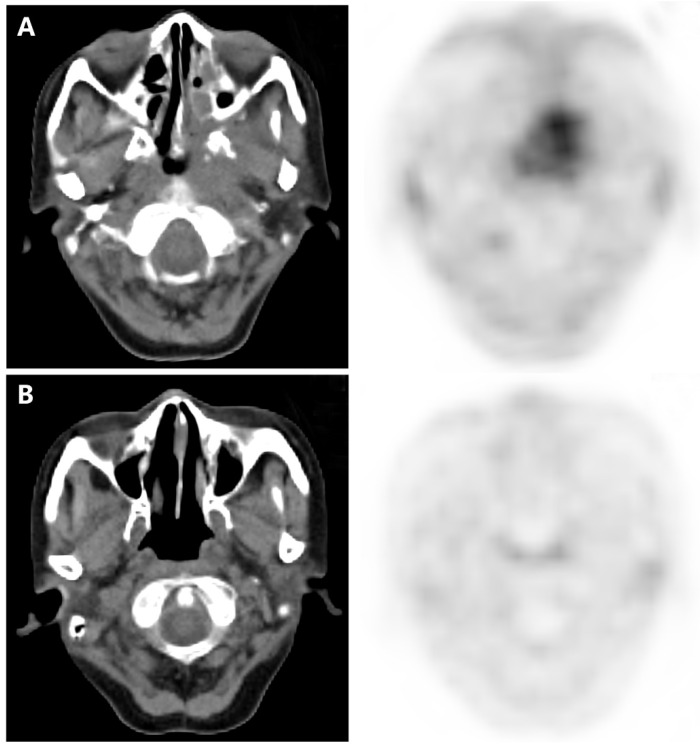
Examples of FLT-PET/CT scans performed at completion of treatment. (A) A rT4N0M0 patient who received FLT-PET/CT scan 4 days after completion of CIRT. Significant FLT uptake was observed, and the SUVmax, MTV and TLT were 2.8, 27.7 and 46.2, respectively. This patient developed local recurrence 6 months after completion of CIRT. (B) A rT3N0M0 patient who received FLT-PET/CT scan 1 day before completion of CIRT. This patient had no residual FLT uptake and remained complete remission till the last follow-up (23 months after completion of CIRT).
